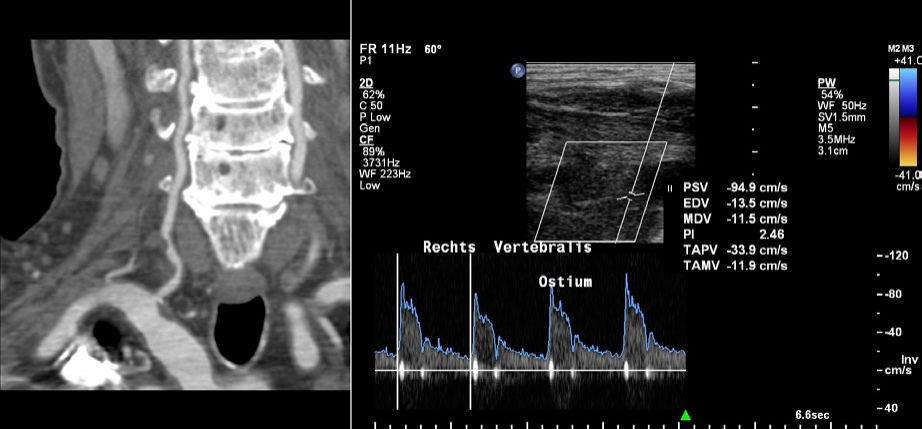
The CT angiography (right) shows a stenosis at the V1 segment. With DUS (left) a normal PSV is found
